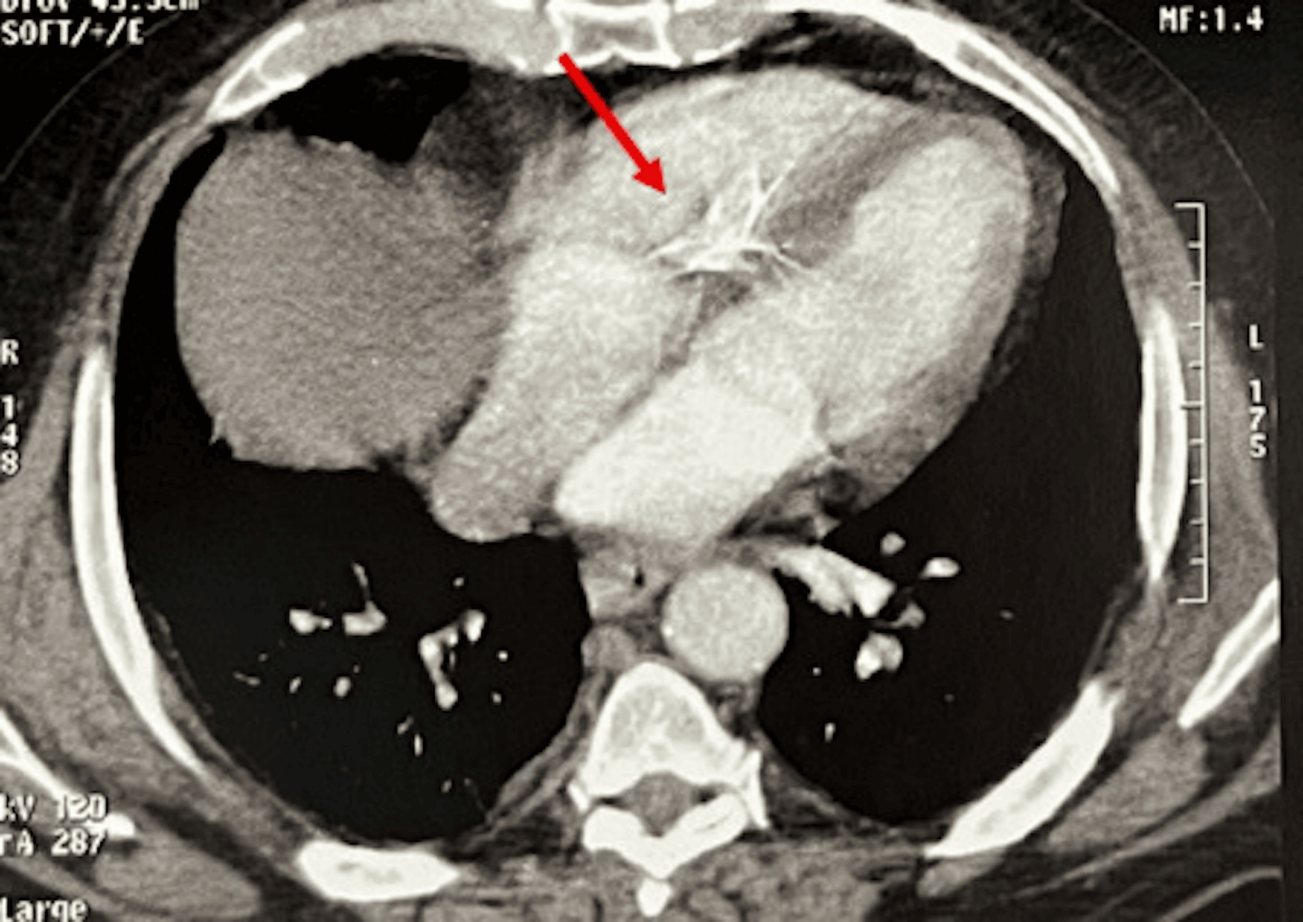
Contrast-enhanced axial CT image demonstrating a metallic structure (red arrow) lodged within the right atrium and right ventricle, consistent with a migrated iliac venous stent.
